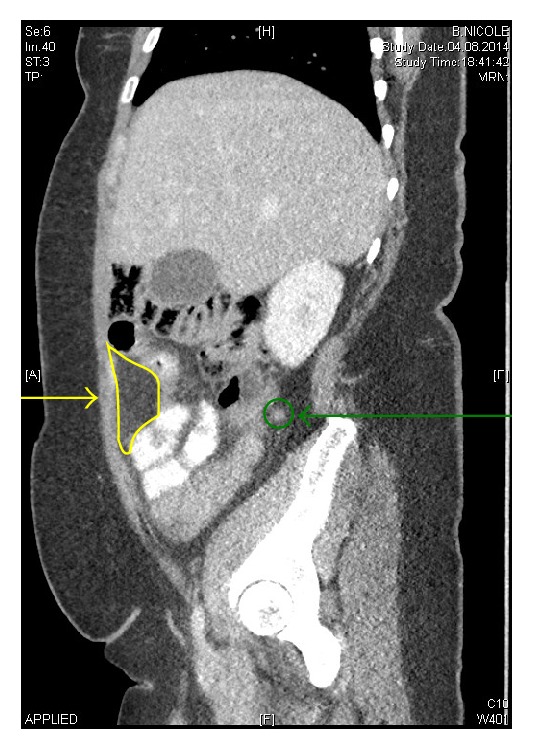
Preoperative CT scan, sagital view; infarcted omentum (yellow line and arrow), appendix vermicularis (green circle and arrow).
